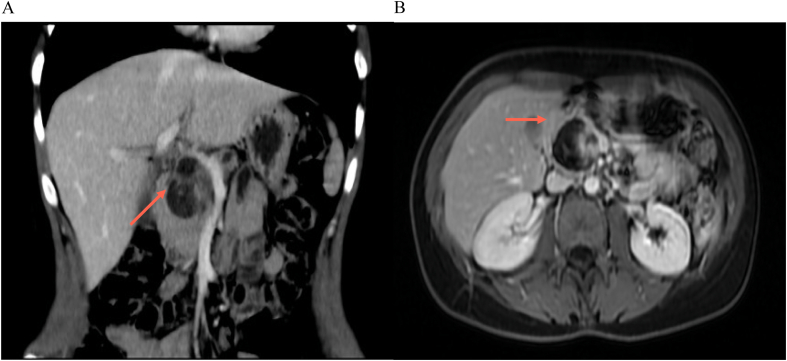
A: MRI in which we observe the cystic multitabulated lesion and the Wirsung duct dilation. B: MRI in which we observe the cystic multitabulated lesion and the Wirsung duct dilation.
